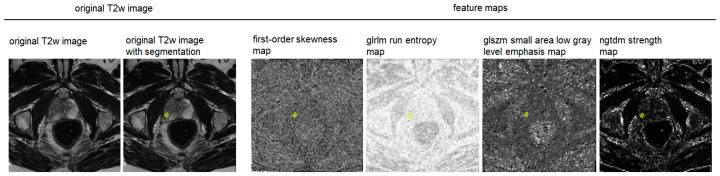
Examples of parametric feature maps. On the left, the original axial T2-weighted (T2w) image and the segmentation, i.e., the volume of interest (VOI, yellow) of the target lesion are shown, and on the right, exemplary parametric feature maps are shown. The feature maps carry the same spatial information as the original image and reflect the feature quantity in brightness. Quantitative information on the feature can be directly drawn from the map once it is computed by extracting the mean. The VOI is copied into the feature maps in an automated step of the software script.
